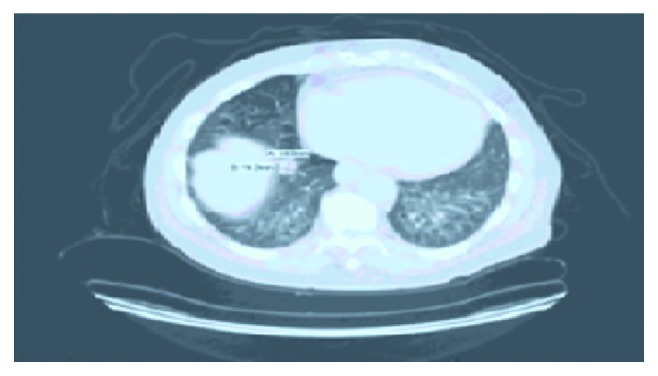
CT chest with contrast showing 1.4 cm right lower lobe lung nodule.
